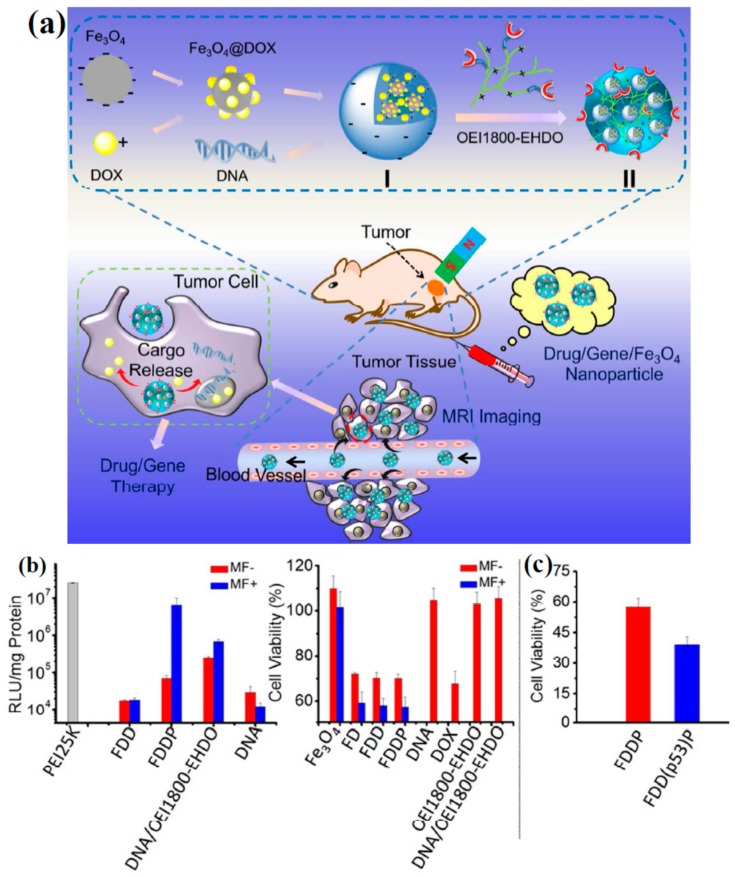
(a) Synthesis process of the Fe_3_O_4_@DOX/DNA/OEI1800-EHDO (FDDP) nanosystem for magnetic targeted drug/gene co-delivery and MRI; (b) transfection activity and cell cytotoxicity of various samples in HeLa cells after 30 min of treatment with/without magnetic field; and (c) cytotoxicity of DOX and pGL-3 co-loaded FDDP NPs and DOX and p53 co-loaded FDD(p53)P NPs in HeLa cells under magnetic field [129].
